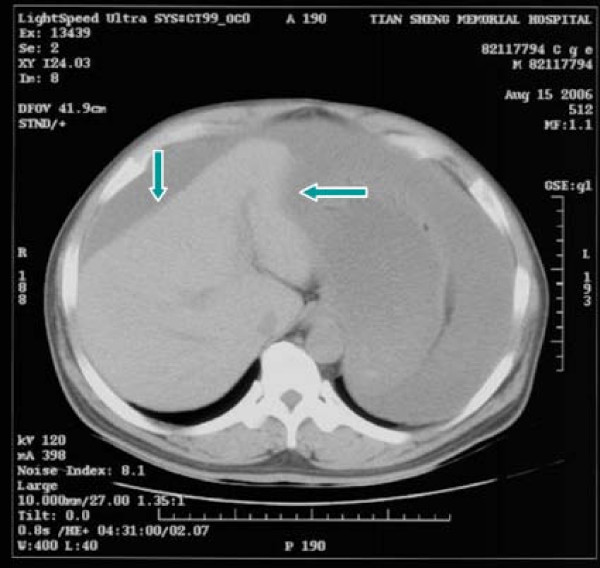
CT scan of abdomen showing pseudomyxoma peritonei with scalloping of hepatic margin (arrows).
